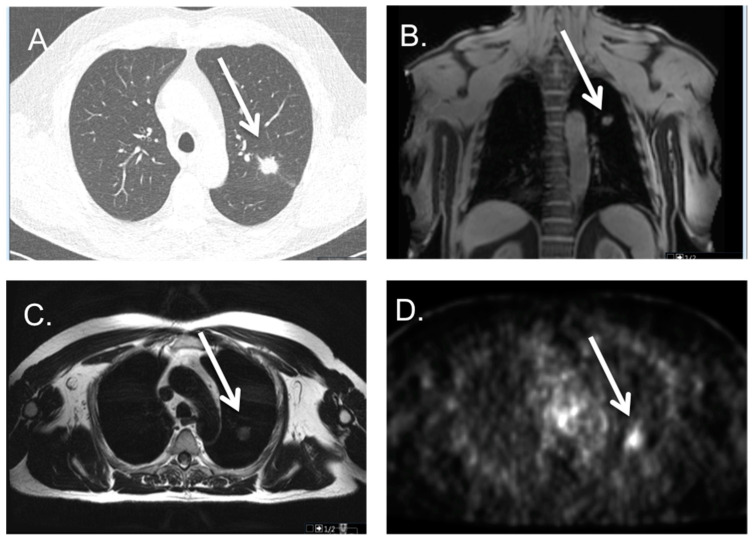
Patient with a primary lung tumor on a PSMA PET-MRI scan; CT and PET-MRI of a patient with spiculated mass in the left upper lobe, visible on CT, see white arrow (A) as well as MRI (STIR coronal (B) and T2 axial (C), see white arrow) and PSMA-PET scan, see white arrow (D). This mass was proven by biopsy to be a primary adenocarcinoma of the lung.
